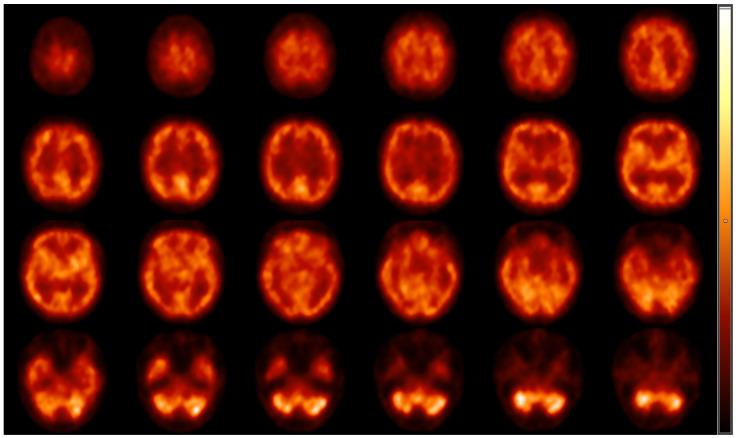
Representative transaxial [^99m^Tc]Tc-HMPAO brain perfusion SPECT images acquired at rest. Following the intravenous administration of 740 MBq of [^99m^Tc]Tc-HMPAO, dynamic SPECT acquisition was performed using AnyScan S Flex SPECT camera (Mediso Ltd., Budapest, Hungary). No perfusion abnormalities can be detected on the slices; the right and the left hemispheres show symmetrical and consistent radiotracer accumulation. High [^99m^Tc]Tc-HMPAO is shown in the cortical grey matter, the region of the basal ganglia and in the visual cortex, while the white matter and the ventricles are presented with faint radioactivity. HMPAO, hexamethylpropylene amine oxime; SPECT, single-photon emission computed tomography.
